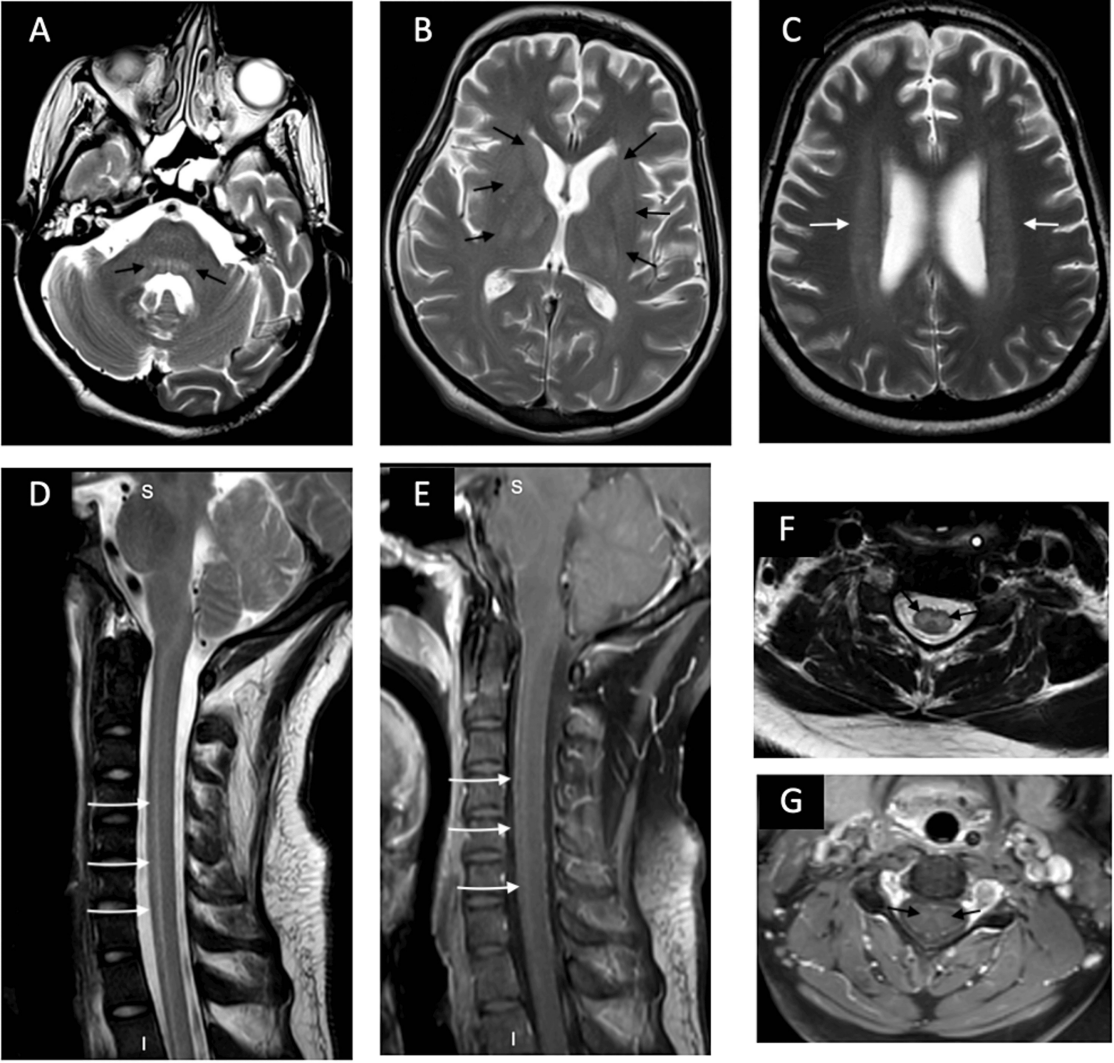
Brain and spinal cord MRI A, B, and C axial T2-weighted images of the head show bilateral symmetrical hyperintensity in the dorsal pons, deep gray matter, and deep white matter (black arrows in A and B, white arrows in C). D and F are sagittal and axial T2-weighted images that show longitudinal hyperintensity mostly involving the central cord, where the gray matter is situated (White arrows in D, black arrows in F). E and G sagittal and axial post-contrast T1-weighted images demonstrate faint contrast enhancement of the same abnormality  (White arrows in E, black arrows in F).
